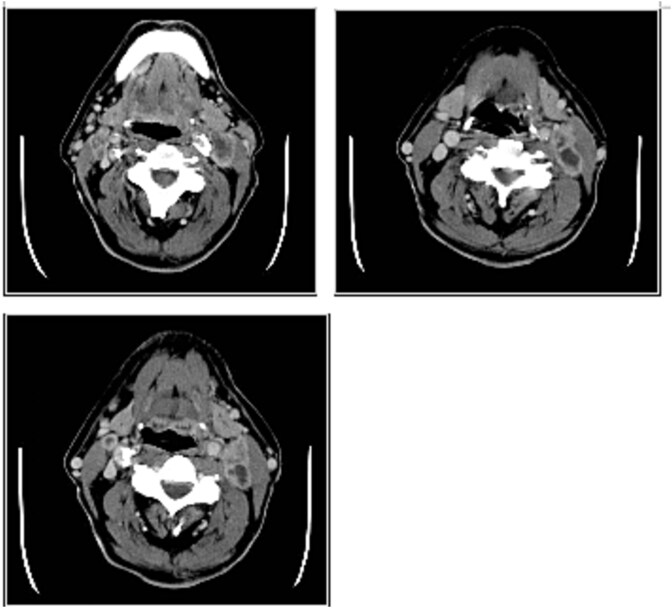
(a, b, c) Axial images in CT of the neck, where the latero-cervical mass is appreciated with impregnation of peripheral contrast medium, uneven with characteristics of malignancy and mimicking a latero-cervical lymphadenopathy.
